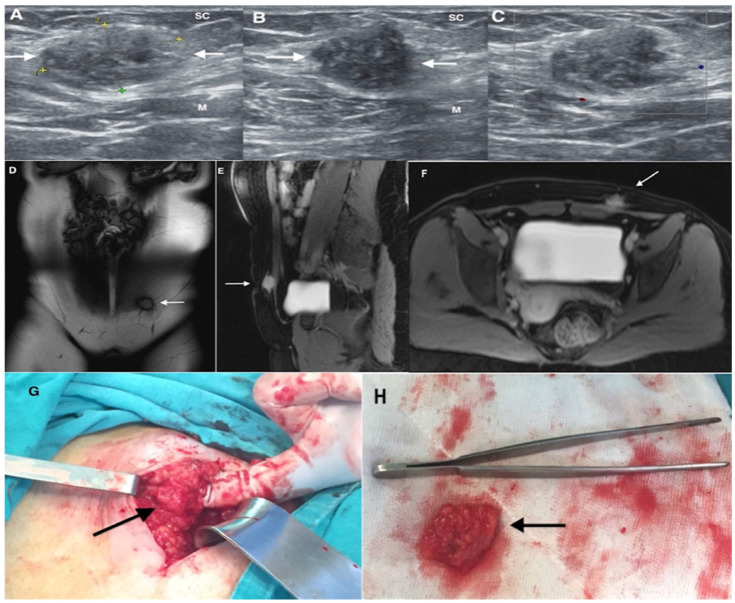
Abdominal wall endometriosis in a 28-year-old woman with a history of one cesarean section presenting with a palpable swelling and cyclic pain at the cesarean scar. (A) Transverse transabdominal ultrasound image shows a hypoechogenic, nodular mass (white arrows) with heterogeneous appearance and ill-defined borders, situated between the subcutaneous tissue (SC) and the rectus abdominis muscle (M). Colored calipers and numbered markers indicate the lesion margins. (B) Longitudinal transabdominal ultrasound image demonstrates the same lesion characteristics. (C) Color Doppler image reveals no vascularity within the lesion; (D) Coronal T2-weighted MRI depicts the lesion at the left corner of the cesarean scar. (E) Sagittal and (F) axial T1-weighted MRI with fat suppression demonstrate high lesion intensity relative to muscle. White arrows in (D–F) indicate the lesion. (G) Wide resection of the endometriotic lesion (black arrow). (H) Macroscopic view of the excised lesion (black arrow).
